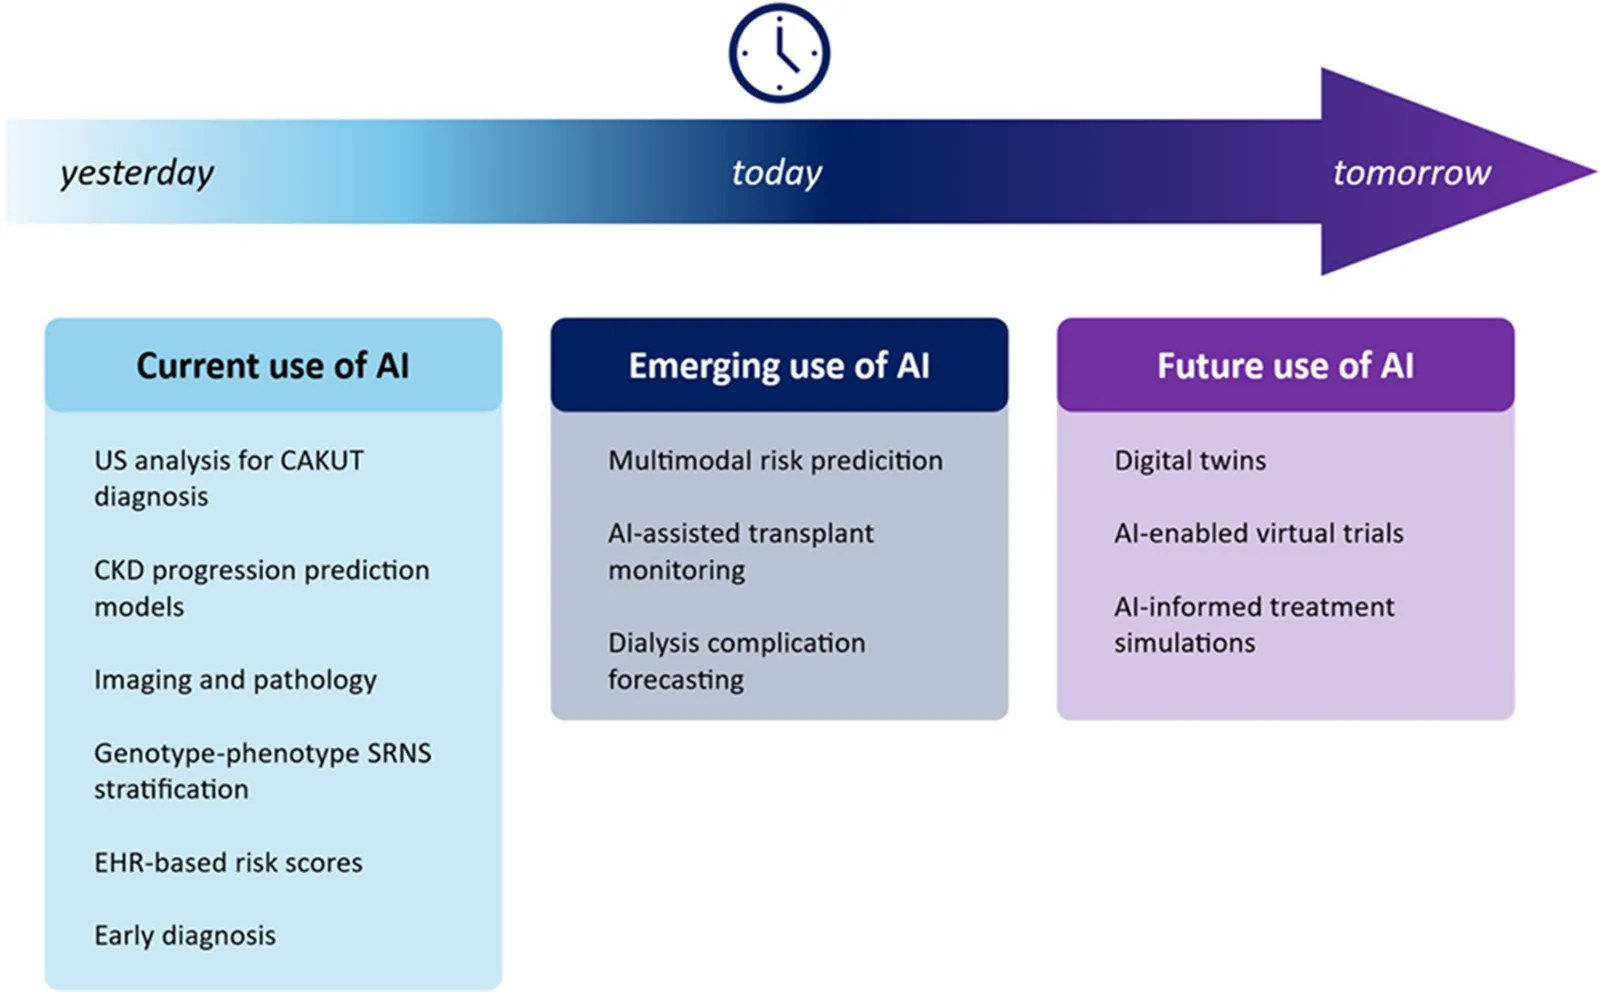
AI applications across the pediatric nephrology care continuum: current and emerging use cases. AI: Artificial intelligence; CAKUT: Congenital Anomalies of Kidney and Urinary Tract; CKD: Chronic Kidney Disease; EHR: Electronic Health Records; SRNS: Steroid-Resistant Nephrotic Syndrome; US: Ultrasound
